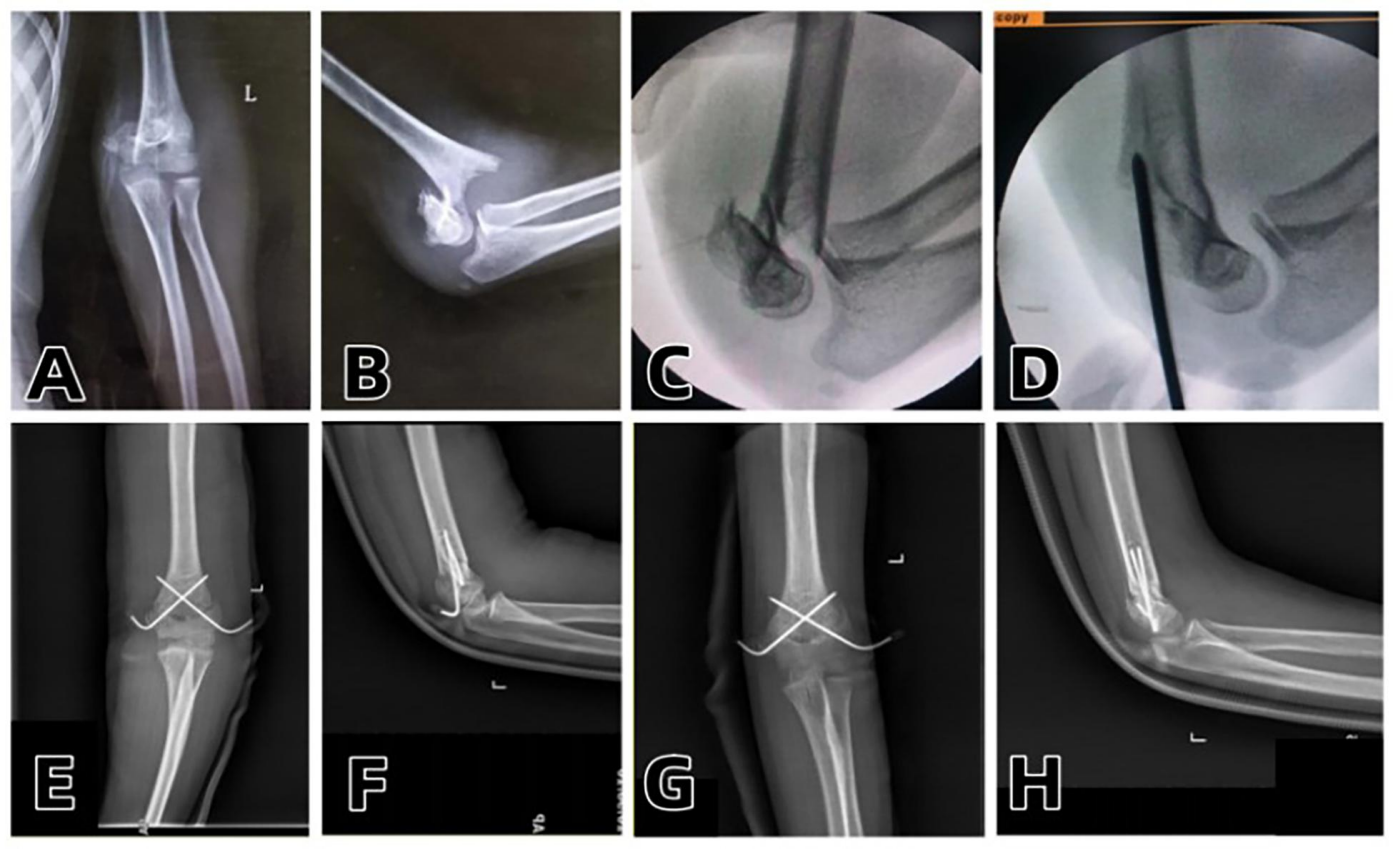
(A,B) Preoperative anteroposterior and lateral radiographs of the elbow joint; (C) Intraoperative failure of manual reduction; (D) Successful intraoperative reduction with a single Kirschner wire prying; (E,F) Intraoperative anteroposterior and lateral radiographs after inserting one Kirschner wire on each of the medial and lateral sides and applying plaster fixation; (G,H) Reexamination at 1 month after surgery showing good fracture healing.
